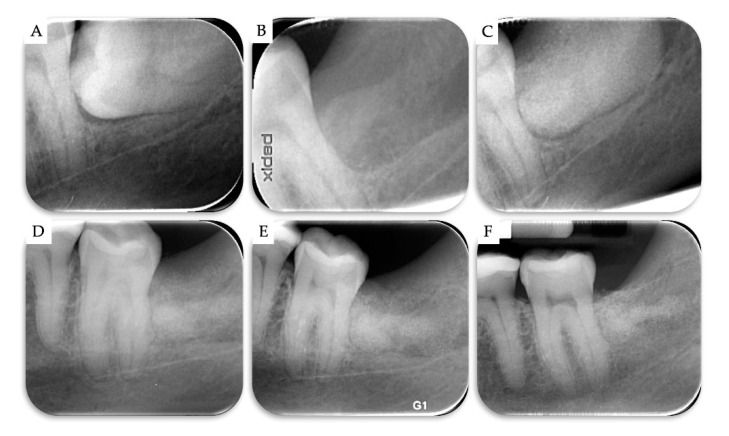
Case no 2. Periapical X-rays (A) at T0, (B) after the surgical removal of the horizontal M3, (C) immediately after grafting the extraction site with β-TCP/CS, (D) 6 months post-operatively, (E) 9 months post-operatively, and (F) 2 years post-operatively.
